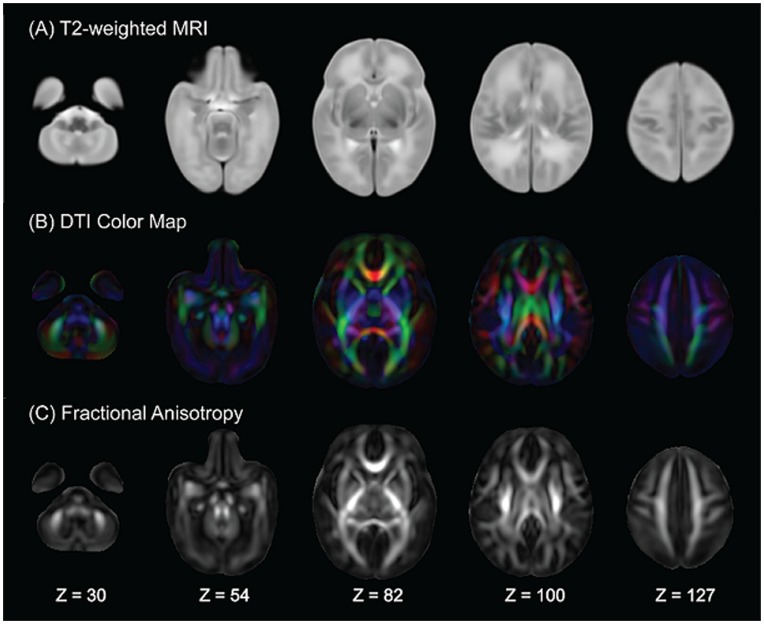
Neonatal structural MRI and DTI atlases. Rows (A-C) respectively show the axial slices of the T2-weighted MRI, the DTI color map and fractional anisotropy (FA) atlases. The anatomical coordinates of individual axial slices are listed on the bottom of the figure.
